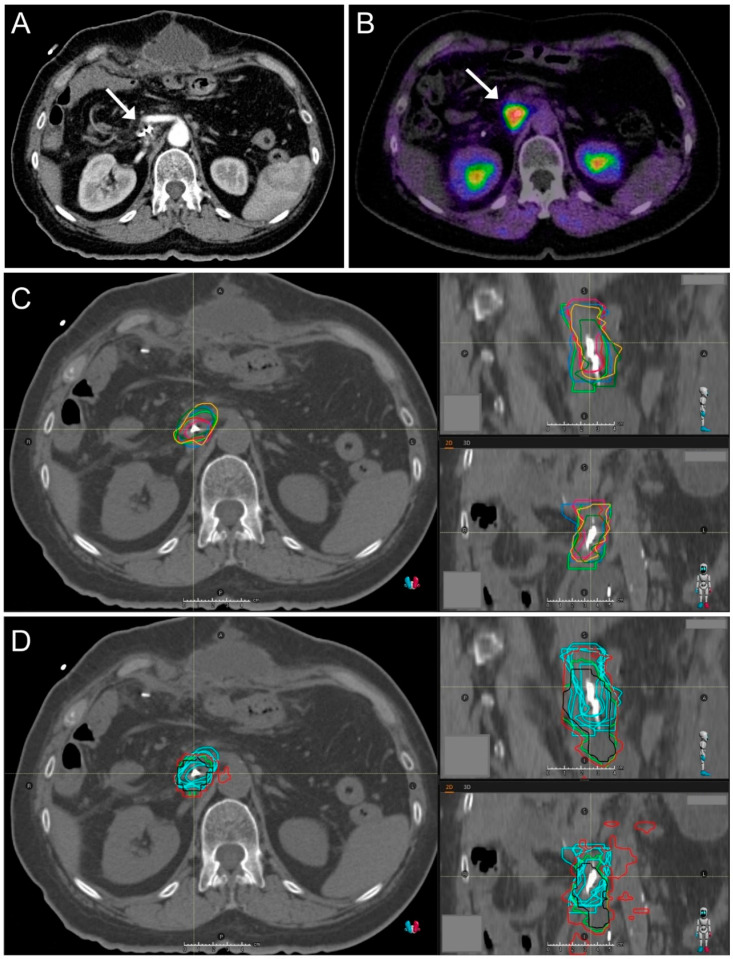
Imaging and target definition of a 66-year old patient with locally recurrent pancreatic cancer showing convincing concordance of FAPI avidity and manually defined cancer tissue. (A) Contrast-enhanced computed tomography (CT) scan. The local tumor recurrence is adjacent to an operation clip, as marked by the white arrow. (B) Fused slice of a fibroblast activation protein inhibitor positron emission tomography (FAPI-PET)/CT scan. The red area represents a high uptake of the FAPI-tracer (white arrow). (C) Gross tumor volumes (GTVs) of six different radiation oncologists, delineated on a conventional CT scan. An axial slice is shown on the left, sagittal and coronal slices are shown on the upper right and lower right, respectively. Each radiation oncologist’s GTV is delineated with a different color. (D) Automated contoured GTVs of three different FAPI thresholds (red: 1.5, green: 2.0, black: 2.5), CT slices arranged as in C. For comparison reasons, the manually defined radiation oncologist’s GTVs of C are demonstrated in light blue.
